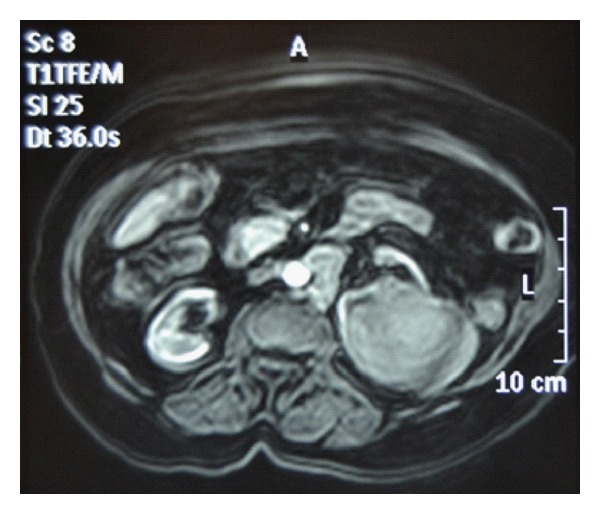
In the MRI, PRL is differentiated from RCC based on the lower signal intensity on unenhanced T1-weighed images compared with a normal renal cortex and fewer enhancements on early gadolinium-enhanced images.
